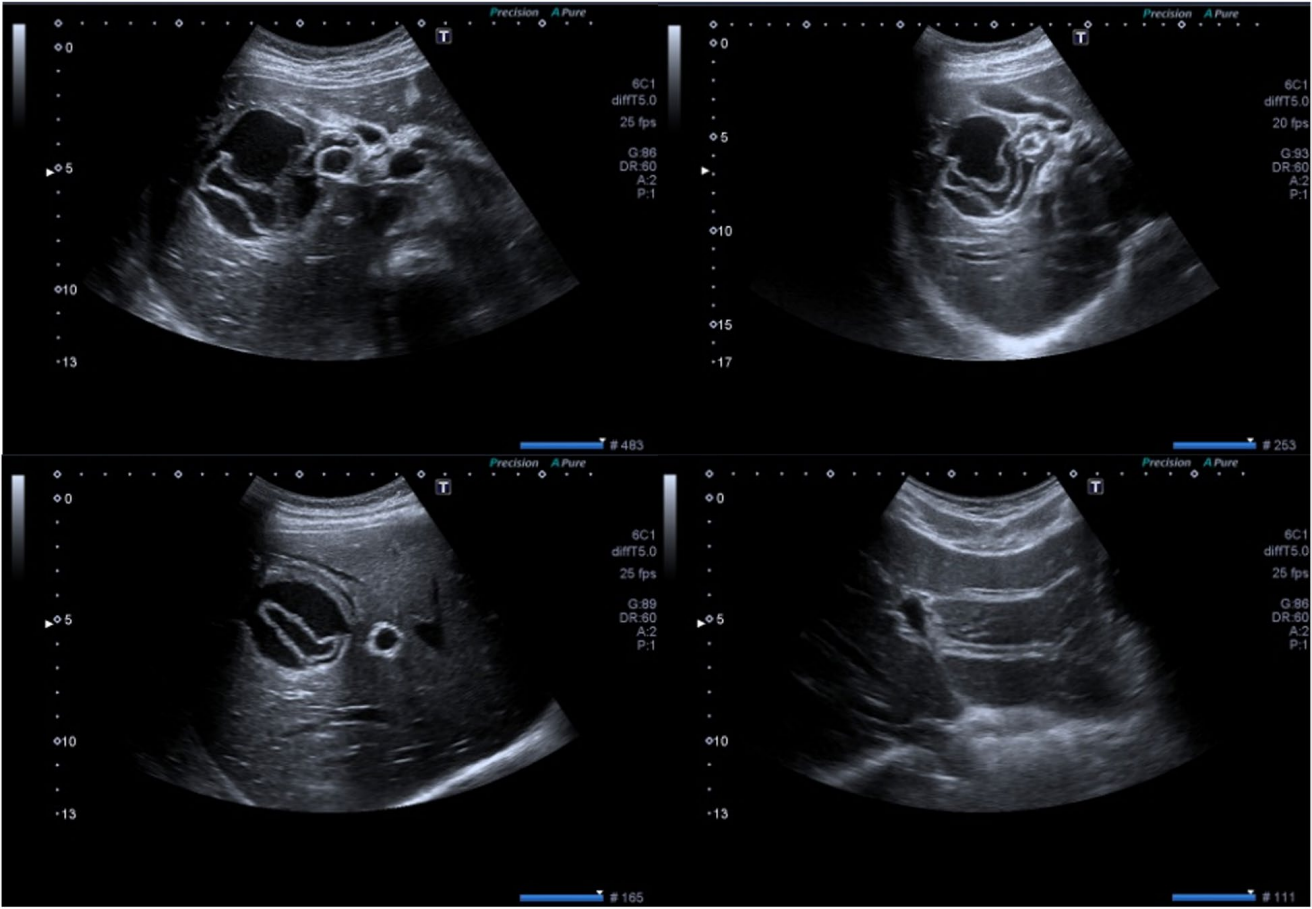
Abdominal ultrasonography images. Serial ultrasonographic images show type 2 ruptured hepatic hydatid cyst, inside of which floating membranes are present, located in segments five and six
